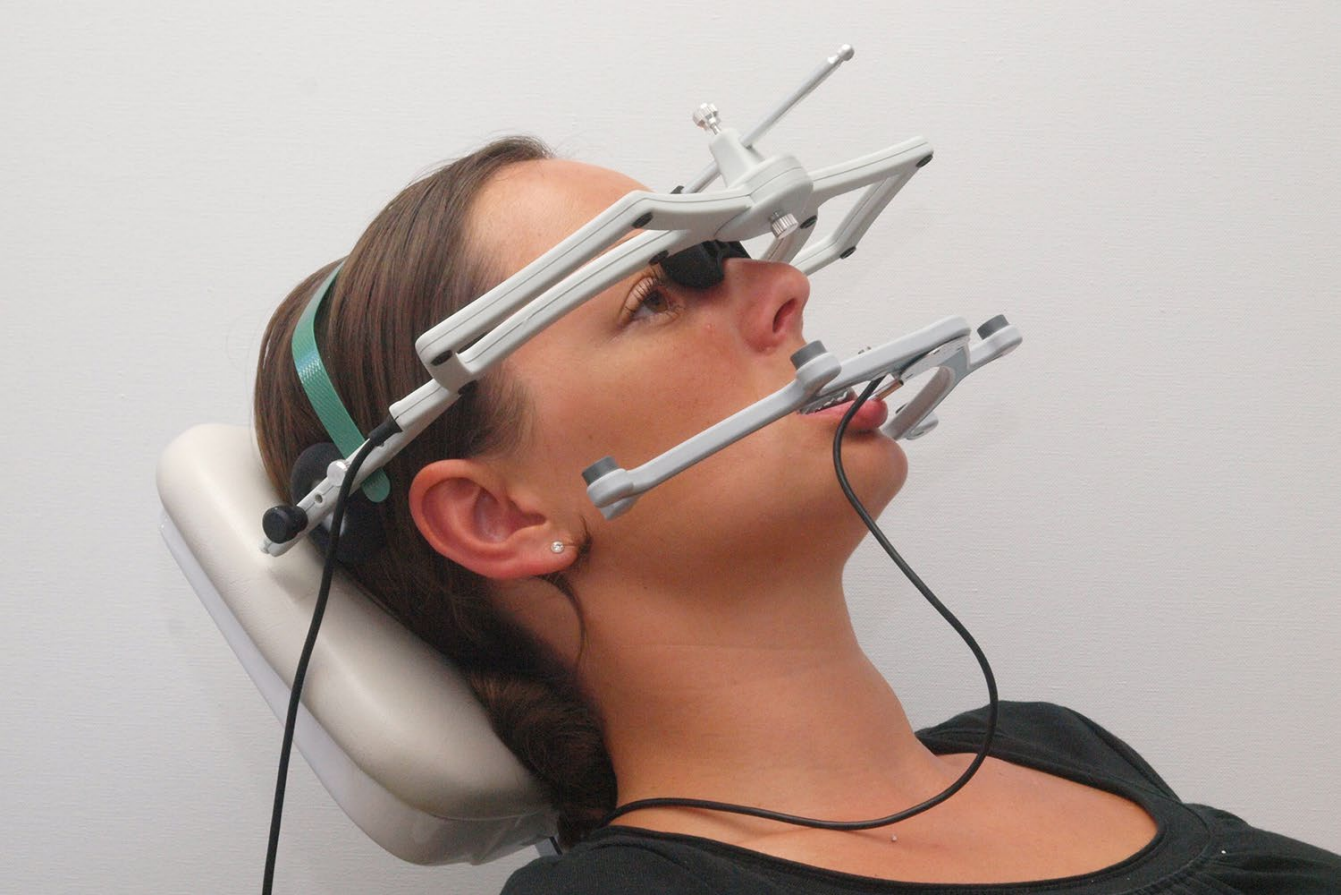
Example of a computer-aided motion recording system based on ultrasonic measurement technology (Zebris Jaw Motion Analyzer+, photo: Ahlers).
